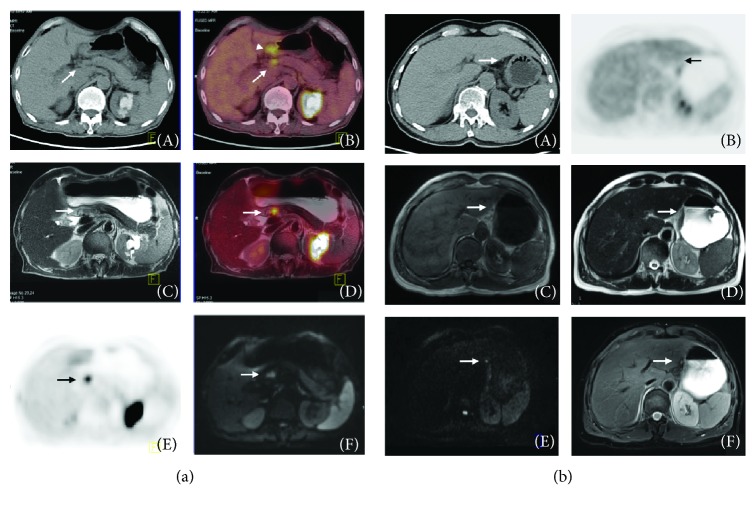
(a) A 75-year-old man with gastric carcinoma diagnosed as lymph node metastasis. (A) The axial CT image shows an enlarged lymph node (arrow) next to the lesion (arrowhead). (B, D) A fusion PET/MRI and PET/CT image shows the enlarged lymph node with FDG uptake and an SUVmax of 3.4 (arrow). (C) One T2-weighted axial image shows a mild-high signal intensity enlarged lymph node (arrow) next to the antrum. (E) One PET image when an obviously avid FDG uptake was observed (arrow). (F) One diffusion-weighted image shows the enlarged lymph node with high signal intensity (arrow), suggesting diffusion restriction. With the aid of these images, indicating a metastatic lymph node, a preoperative diagnosis of N+ could be made. (b) A 67-year-old man with gastric carcinoma diagnosed as a short diameter 5 mm perigastric metastatic lymph node (arrow). The lymph node near the lesser curvature of the stomach is not found as a metastasis lesion on the CT and PET (A, C, D) and no increased FDG uptake (B). It can be diagnosed through diffusion-weighted imaging (*b* = 800) and T2-weighted saturated fat imaging due to its high signal intensity on both modalities (E, F).
